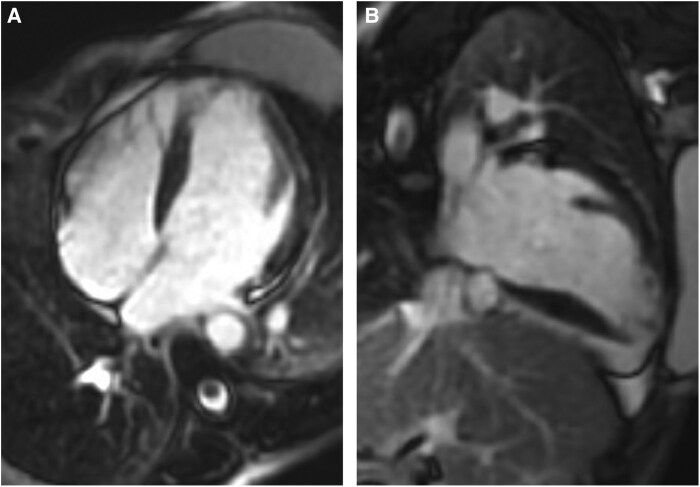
Cardiovascular MRI. (*A*) and (*B*) showing late-gadolinium enhancement (LGE) in the inferior and inferoseptal segments of the akinetic LV apex, suggestive of myocardial infarction.
